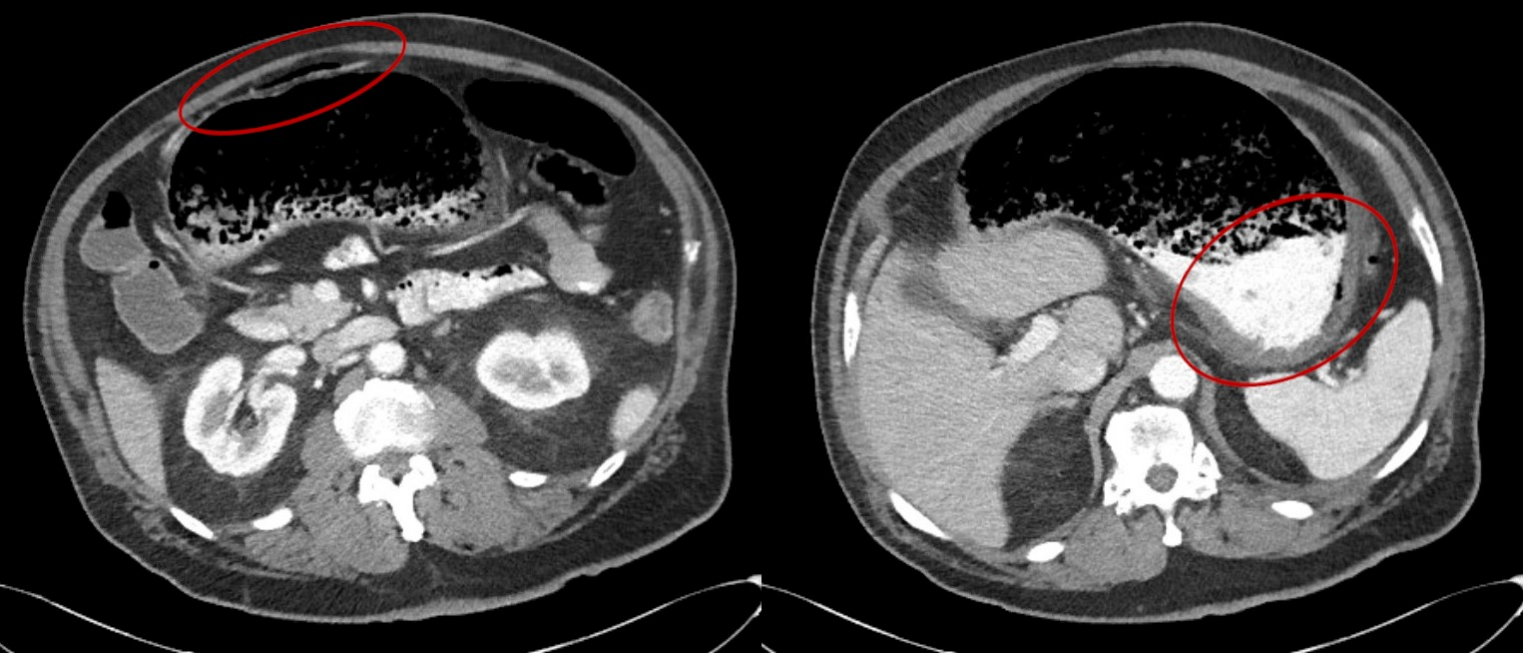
CT Abdomen showing gastric wall edema and gas in the peri-gastric vessels, some of which is highlighted in red.
